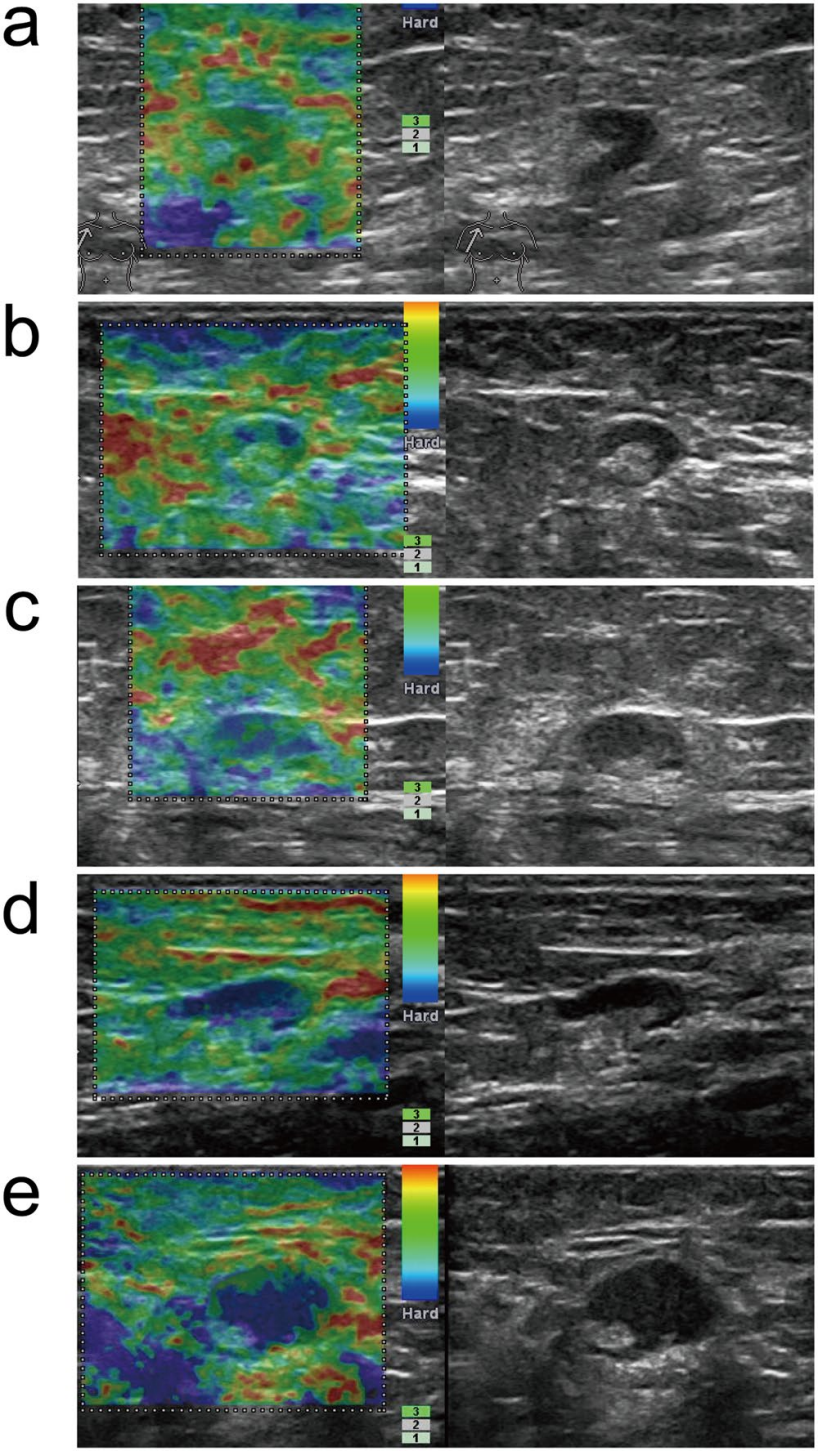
Gray-scale-RTE dual-mode ultrasonogram of Pattern I ALNs. Images present the general appearance of lesions for RTE scores of (a) 1, (b) 2, (c) 3, (d) 4, and (e) 5 of the lymph nodes with hila: (a) Green portion occupying almost all of the cortex; (b) Blue portion occupying less than 50% of the cortex; (c) Blue portion occupying more than 50% of the cortex, with a scattered green/red portion; (d) Blue portion occupying almost all of the cortex; (e) Blue portion occupying almost all of the cortex, with a green/red ring on the edge of the node.
